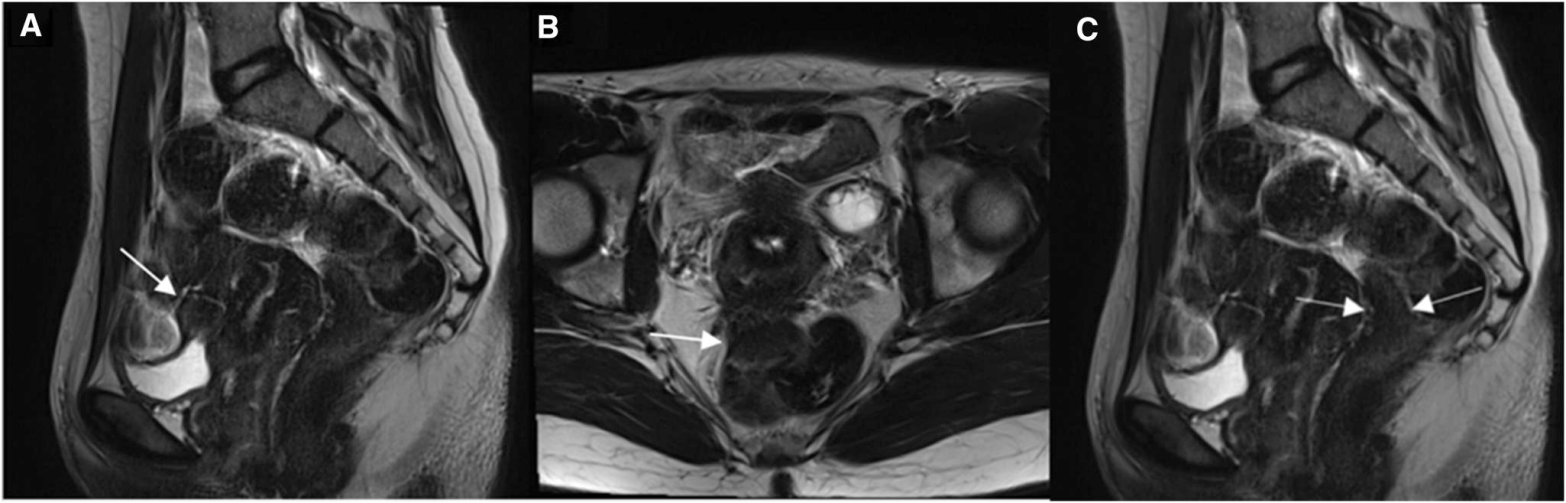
A 32-year-old female with history of DE (Case courtesy of Dr. Laura Fender). MRI pelvis showed (A) endometriotic nodule in the vesicouterine fold (arrow), (B and C) a large mushroom-shaped bowel DE nodule which is tethered to the posterior cervix (arrows).
